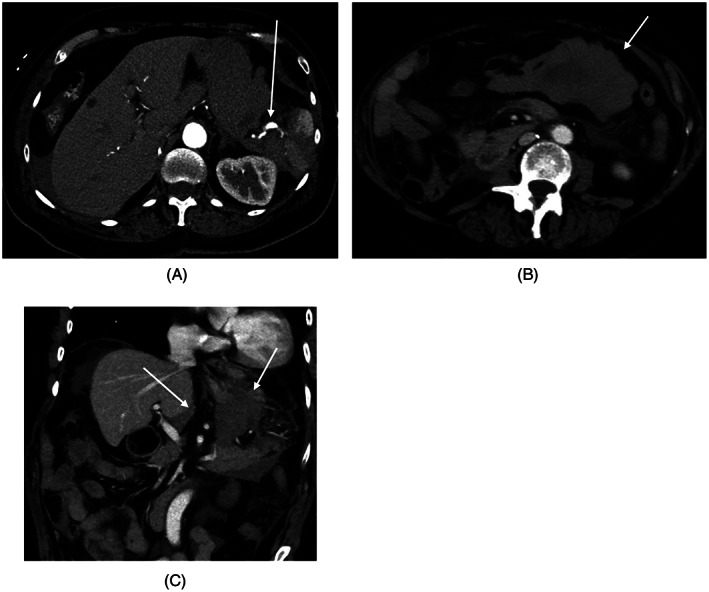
CT SCAN with contrast acquired March, 2020 to investigate patient's left upper quadrant pain: (A) Axial CT SCAN shows ruptured splenic artery aneurysm (arrow) with perisplenic hematoma and hemoperitoneum; (B) Axial CT SCAN shows hemoperitoneum in the lesser sac (arrow) due to ruptured splenic artery aneurysm; (C) Coronal CT SCAN shows hemoperitoneum in the lesser sac (arrow) due to ruptured splenic artery aneurysm
